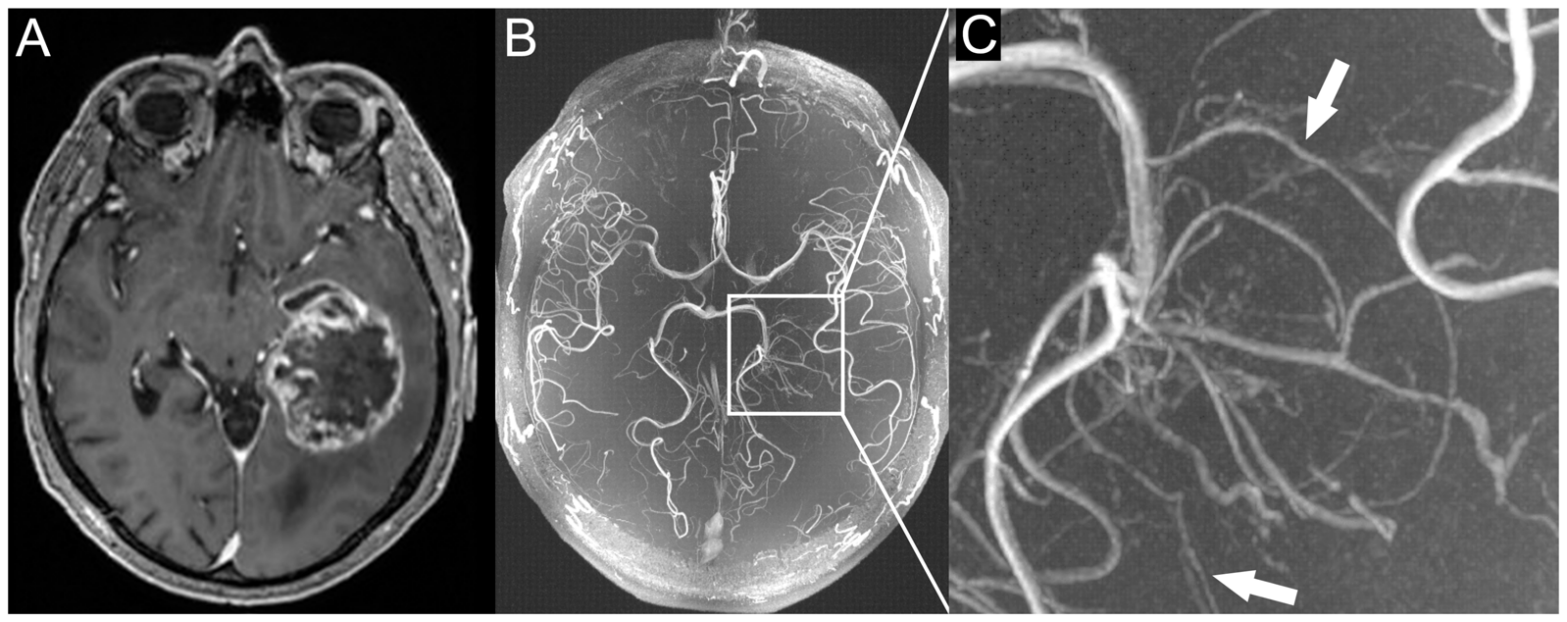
Patient with a left temporal glioblastoma. A) contrast enhanced T1-weighted images at 3 Tesla. B) 7 Tesla ToF angiography maximum intensity projection; enlarged in C). Tumor vessels with multiple branches can clearly be identified within the glioblastoma (white arrows in C). Signal intensity in the tumor vessels is reduced compared to the regular vessels due to signal loss in the irregular and leaky tumor vessels.
